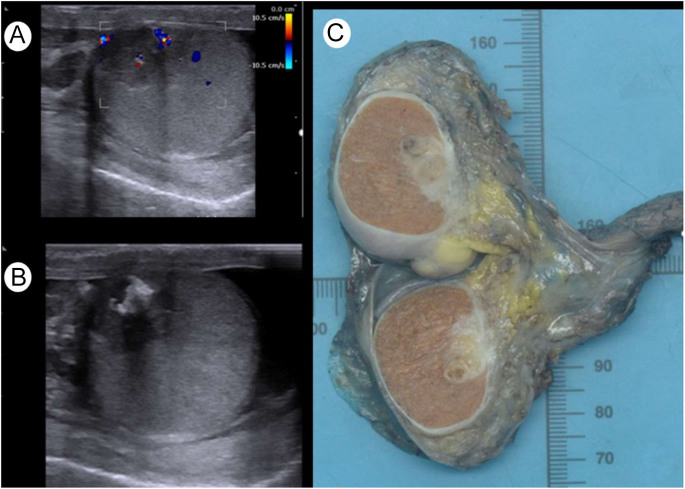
Ultrasound images of the testicular tumour of patient 2 upon presentation (A and B) and macroscopic image of the tumour after surgical resection (C).
